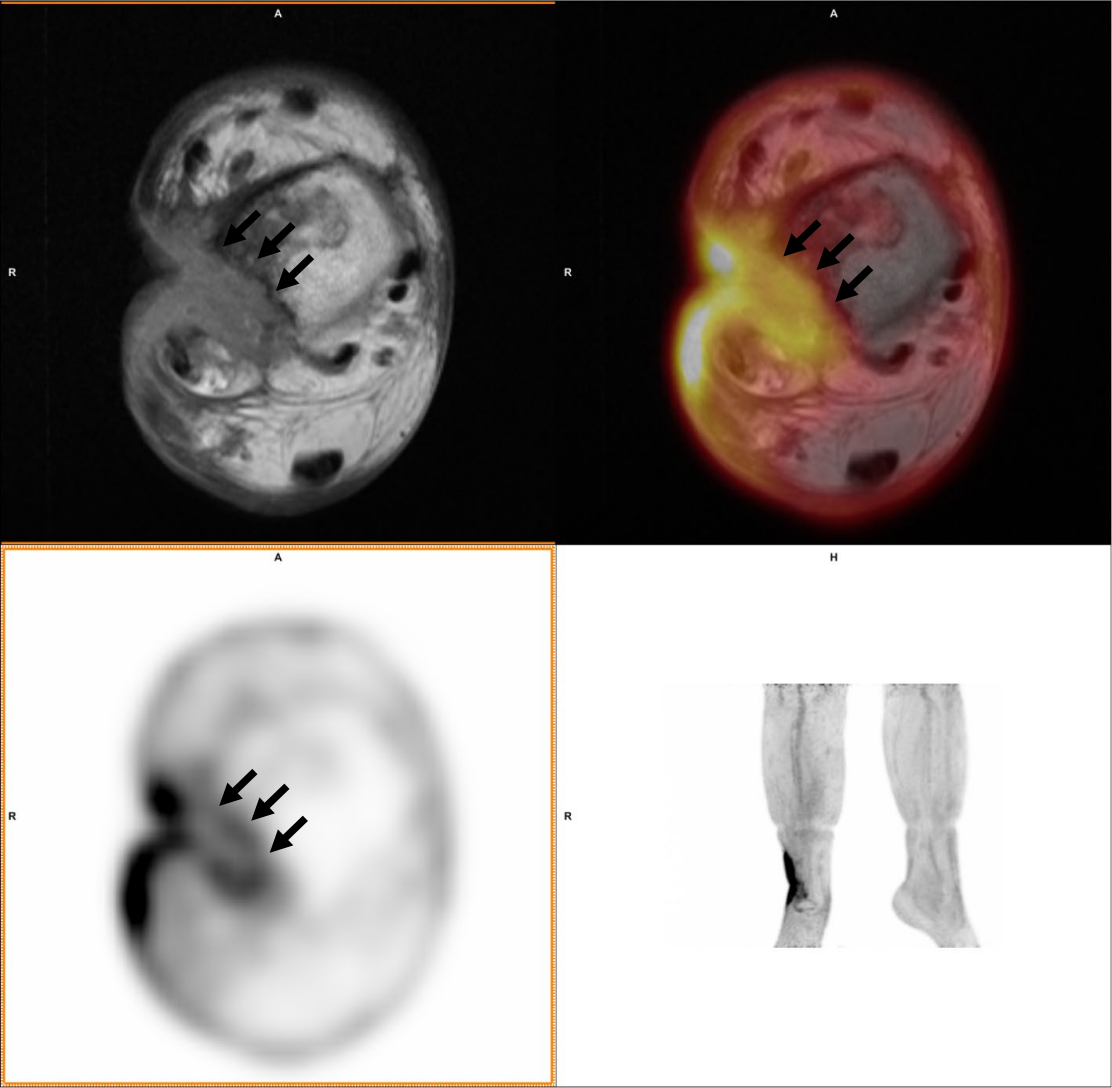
A 65 year old woman with a 4 year old bone injury. Based on MRI, induration of soft tissue was found and the radiologist assessed that the ossal defect was pre-existing with only soft tissue remaining in the defect, leading to the conclusion of soft tissue infection. PET did reveal increased FDG uptake down to the bone (arrows). In the consensus reading this was concluded to be osteomyelitis. The final clinical diagnosis was (culture-negative) osteomyelitis based on the perioperative experience and over 3 years follow-up
